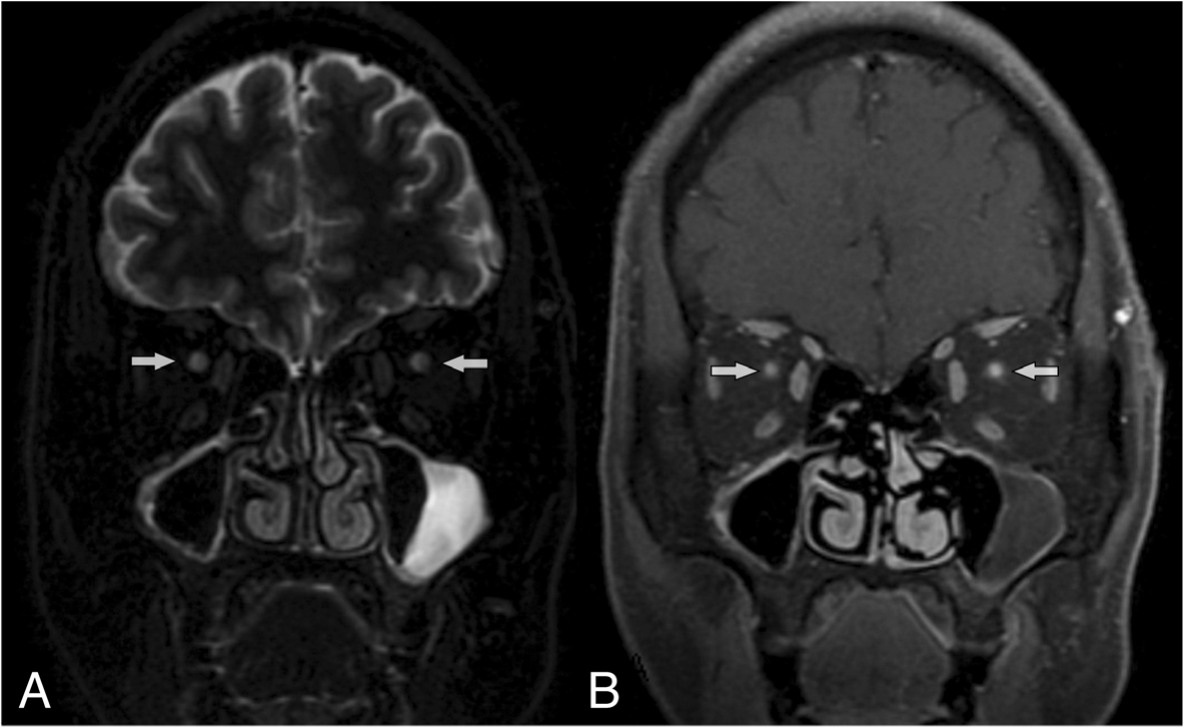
Coronal optic nerve MRI for patient 8. a sequence (T2-weighted imaging) showing hypersingnals in both optic nerves. b sequence (T1-weighted imaging with contrast and fat suppression) showing enhancement of both optic nerves
